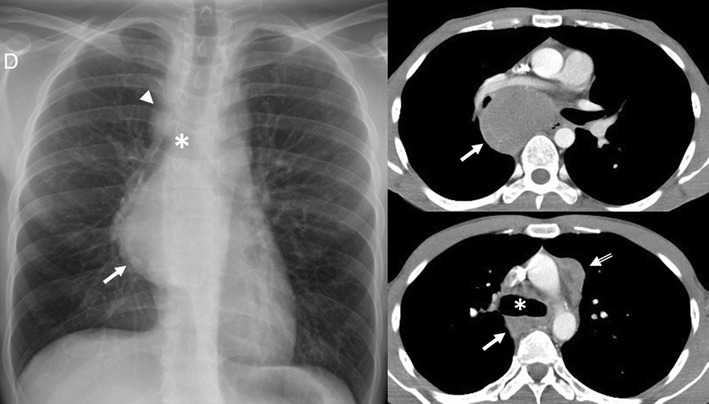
A 28-year-old man with Hodgkin lymphoma. Frontal chest radiograph and contrast-enhanced CT scan show a homogeneous soft tissue mass at the level of the subcarina (*arrows*). An aortopulmonary window lymphadenopathy can be detected on CT scan (*open arrow*). The right paratracheal stripe is not seen on frontal chest radiograph, having been most probably obliterated by a right paratracheal lymphadenopathy (*arrowhead*). * Carina
